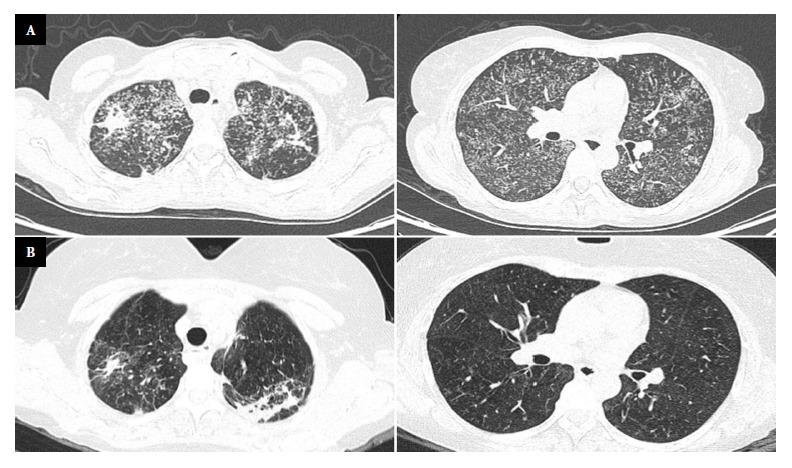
Computed tomography before and after initiation of treatment.
